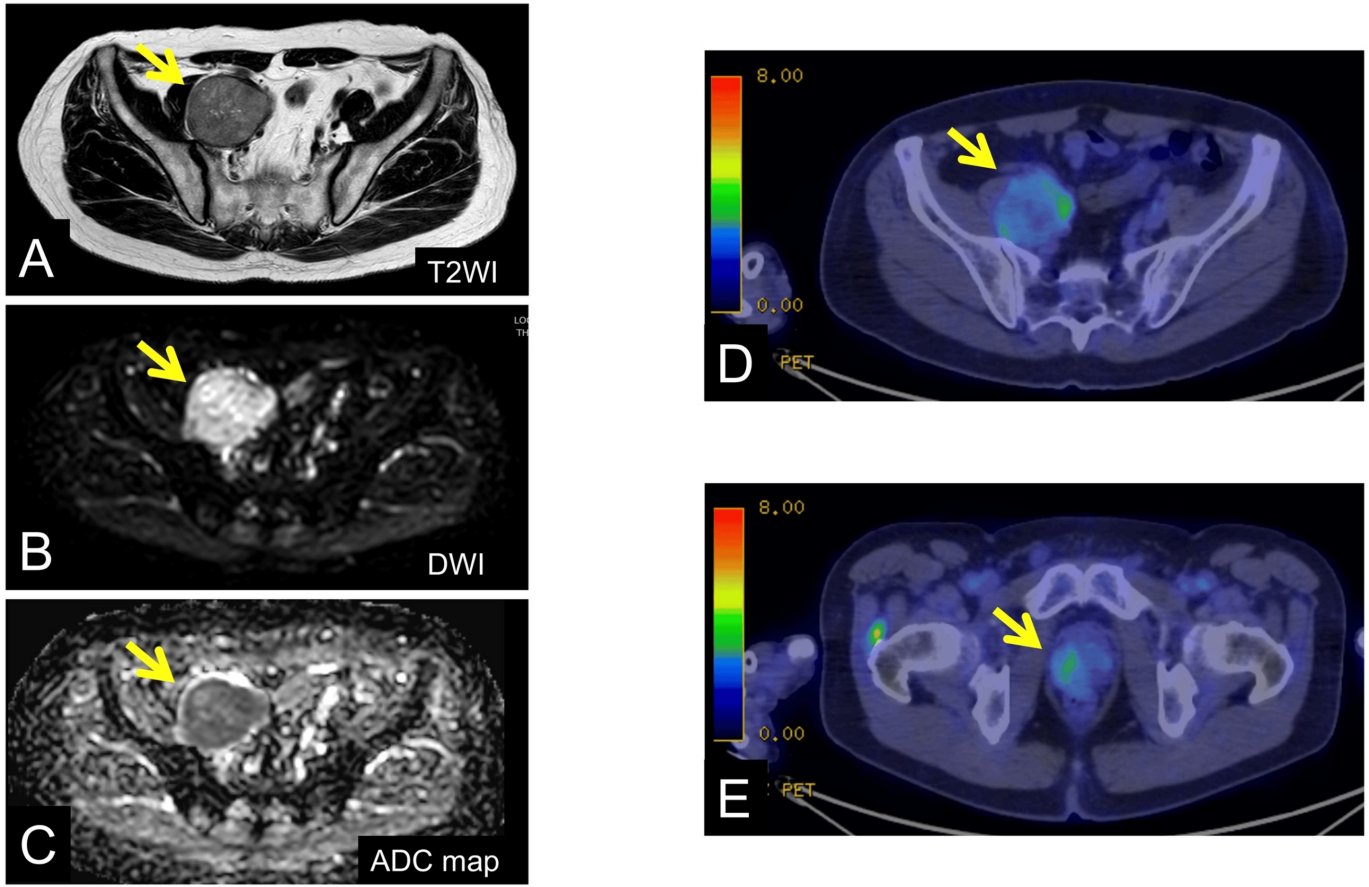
MRI findings and FDG PET-CT images A-C: MRI findings: The yellow arrow indicates the right pelvic lymph node. The lymph node shows no communication with adjacent vessels on T2WI, high signal intensity on DWI, and low signal intensity on the ADC map. D and E: FDG PET-CT images: The yellow arrow indicates (D) the right pelvic lymph node and (E) the right peripheral zone of the prostate, showing increased uptake (SUVmax: 4.4 early/4.7 delayed in the lymph node; 3.5 early/4.2 delayed in the prostate). MRI: magnetic resonance imaging, FDG: fludeoxyglucose, PET-CT: positron emission tomography-computed tomography, T2WI: T2-weighted imaging, DWI: diffusion-weighted imaging, ADC: apparent diffusion coefficient, SUVmax: maximum standardized uptake value
